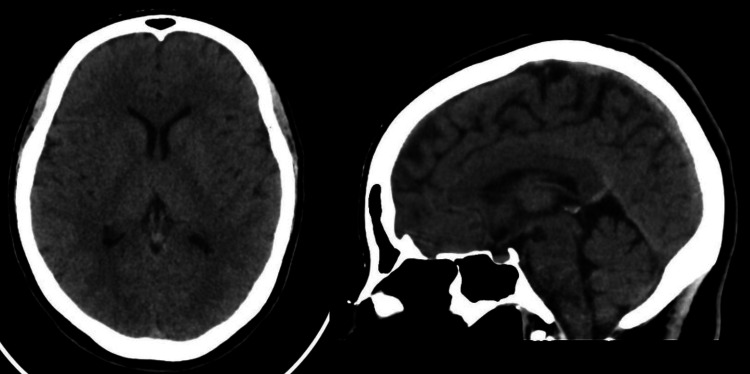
Axial non-contrast CT of the brain at the level of the basal ganglia and midline sagittal CT image showing normal finding
